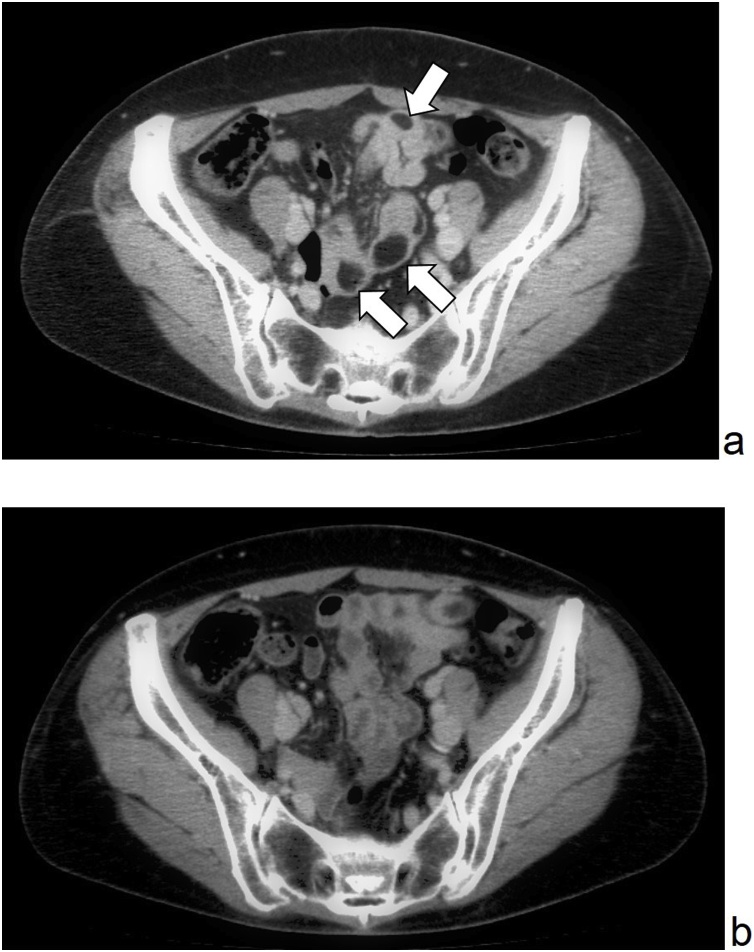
(a) Diffuse multiple lipoma (allow) was found from the proximal jejunum to distal ileum in pre-operative CT.(b) No obvious recurrence or increase of lipoma have been observed in post-operative follow-up CT.
